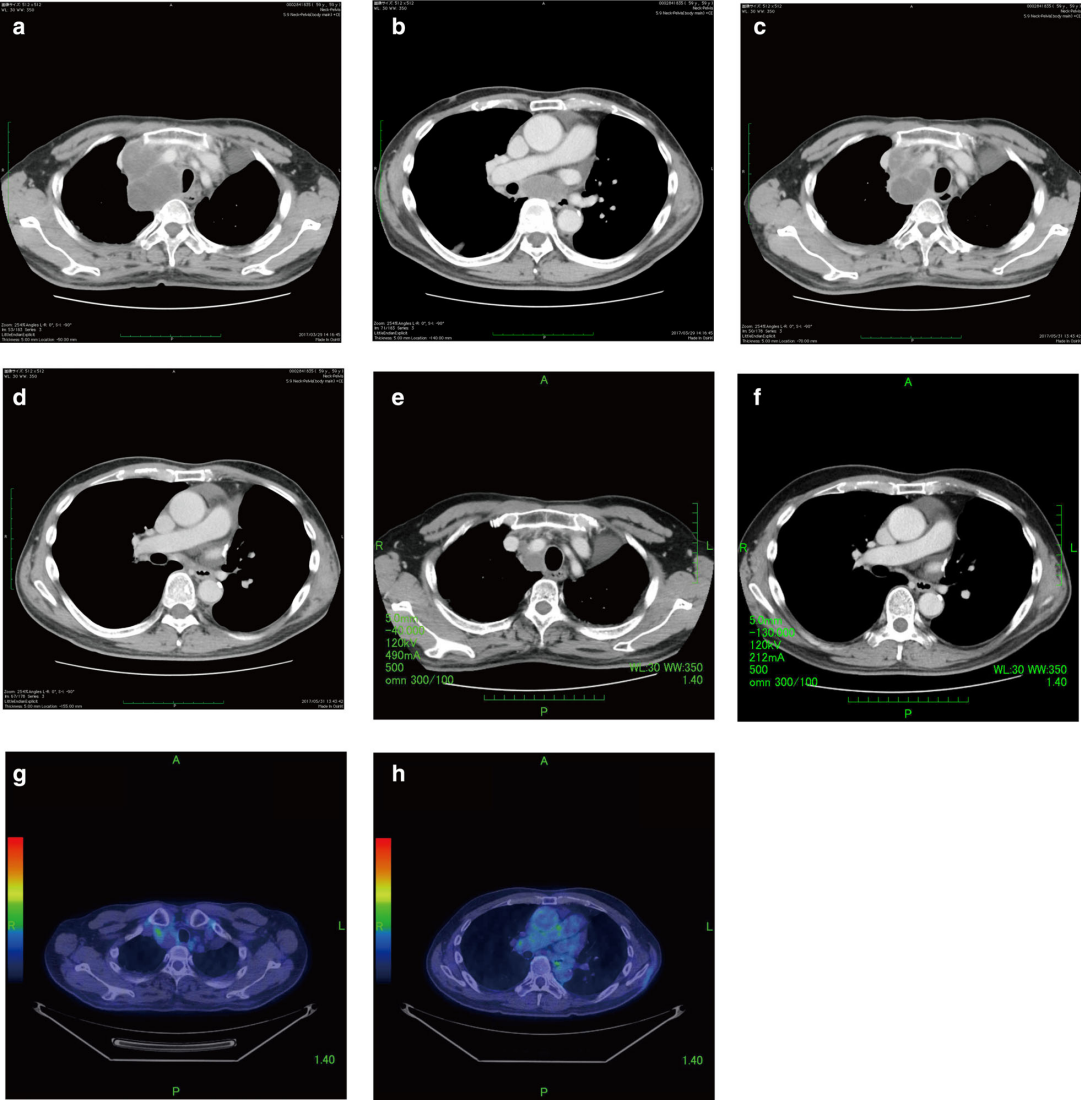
Clinical course: treatments, chest computed tomography (CT), and positron emission tomography (PET)‐CT. (a) Before pembrolizumab administration, CT shows superior mediastinal lymph node swelling. (b) Before pembrolizumab administration, CT shows lower mediastinal lymph node swelling. (c) After three cycles of pembrolizumab, CT shows reduction of the superior mediastinal lymph node. (d) After three cycles of pembrolizumab, CT shows reduction of the lower mediastinal lymph node. (e) After 24 cycles of pembrolizumab, CT shows reduction of the superior mediastinal lymph node. (f) After three cycles of pembrolizumab, CT shows reduction of the lower mediastinal lymph node. (g) PET‐CT 24 cycles after pembrolizumab shows a pronounced decrease in tracer uptake in the superior mediastinal lymph node. (h) PET‐CT 24 cycles after pembrolizumab shows a pronounced decrease in tracer uptake in the lower mediastinal lymph node
